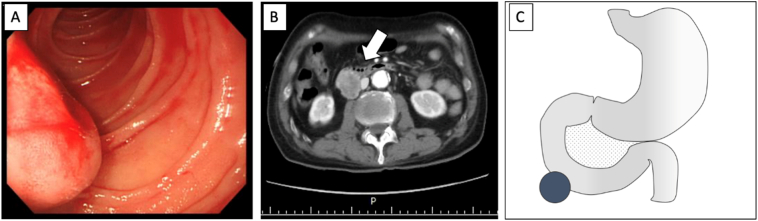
Tumour location and findings in patient one. (A) Endoscopic findings shows bleeding from the apex of the tumour in the 2nd portion of the duodenum. (B) An abdominal enhanced CT scan show a 2.5-cm-sized enhanced tumour between the IVC and the duodenum. Arrow, tumour. (C) Scheme for the site of the tumour. •, tumour. Abbreviations: CT, computed tomography, IVC, inferior vena cava.
